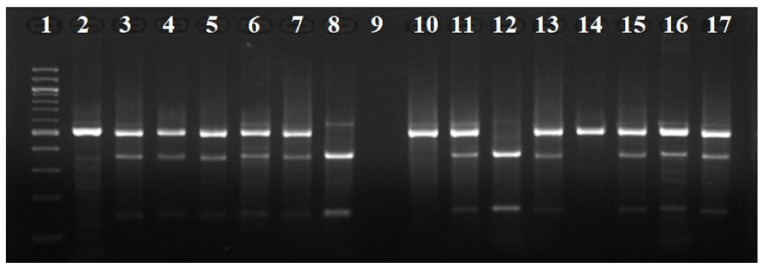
Agarose gel electrophoresis of the digestion products of the 677C>T polymorphism of the *MTHFR* gene from our study. Lane 1: molecular weight index (100 bp ladder). Lanes 2, 10, 14: samples homozygous for the C allele (CC). Lanes 8, 12: samples homozygous for the T allele (TT). Lanes 3, 4, 5, 6, 7, 11, 13, 15, 16, 17: samples heterozygous (CT). Lane 9: No samples were used.
